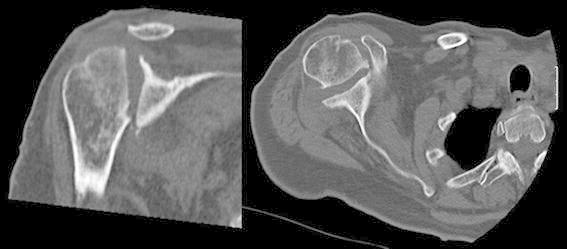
CT-scan images showing marked glenohumeral destruction, humeral head flattening, and articular narrowing
